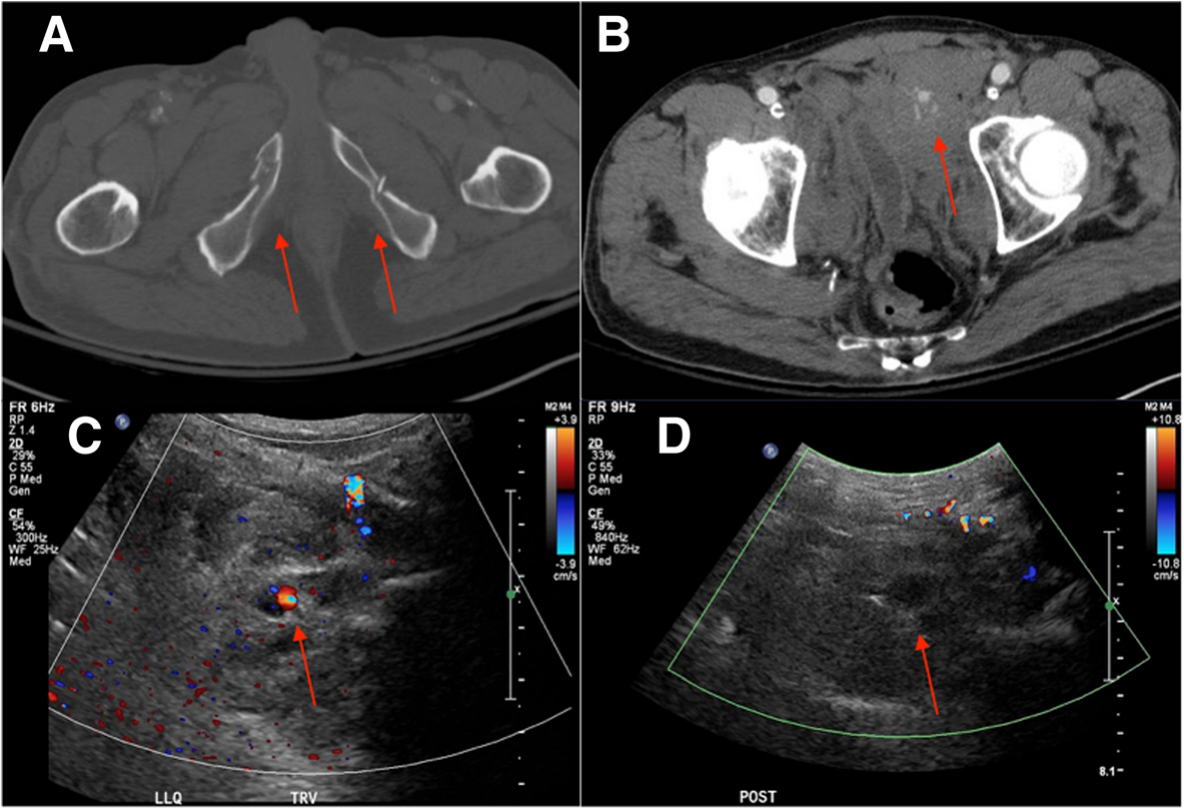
a and b Contrast enhanced CT of the pelvis demonstrates fractures of the bilateral pubic rami and areas of active hemorrhage likely arising from left external pudendal artery. c Color Doppler ultrasound image through the prevesical hematoma demonstrates focal pseudoaneurysm formation corresponding to the area of extravasation on the CT. d Following injection of 2500 units of thrombin, no residual vascular flow was identified in the pseudoaneurysm
